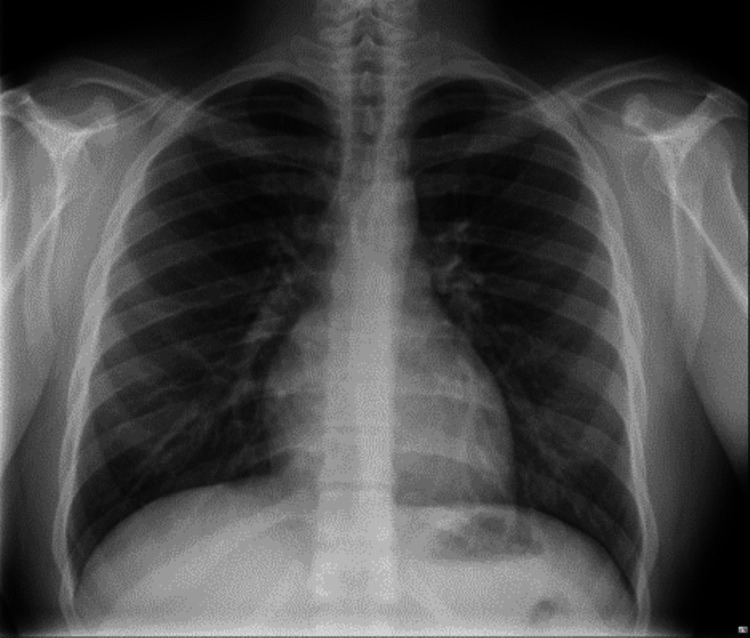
Initial chest X-ray from the emergency department. No acute findings were reported.
